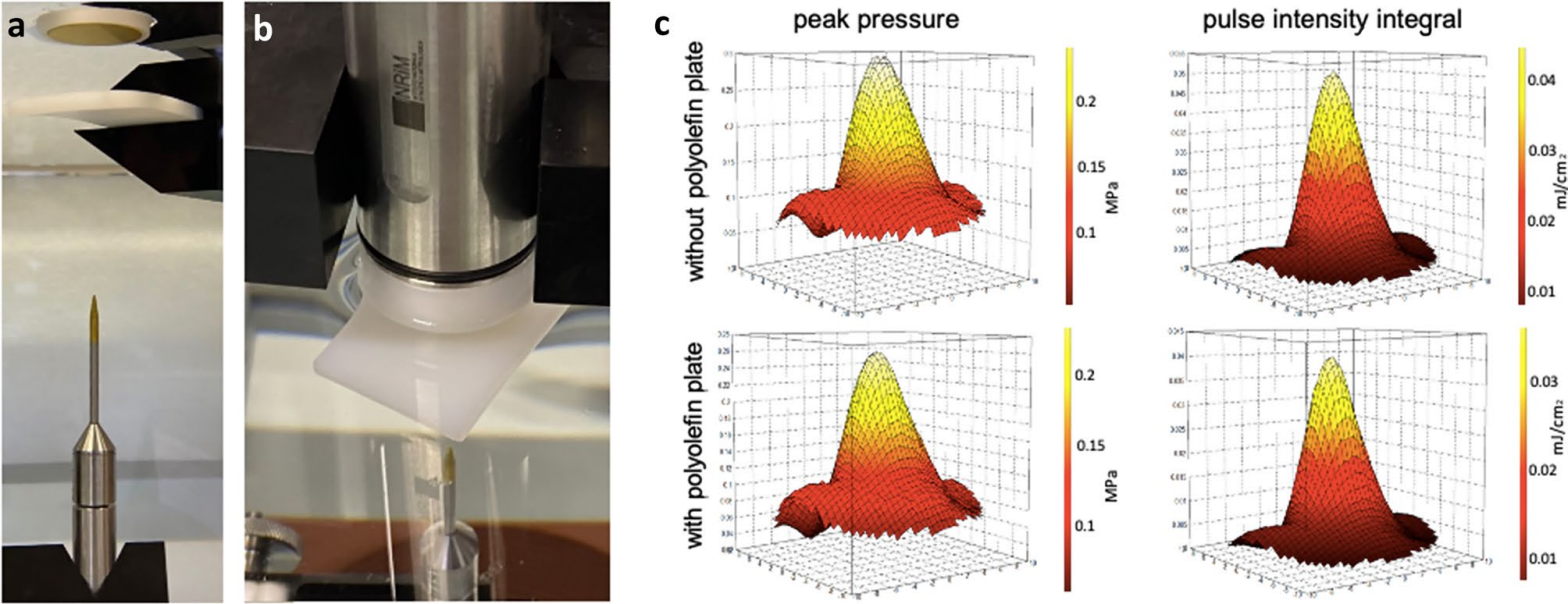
Acoustic characterization of the ultrasound-transparent polyolefin plate. (a,b) Measurements setup: (a) from top to bottom: surface of TRA08 transducer, polyolefin plate, HNC-0400 hydrophone. (c) Comparisons between two-dimensional measured array pressure (left panels) and energy (right panels), with polyolefin plate placed at 15 mm from the transducer surface (lower panels) and without polyolefin plate (upper panels).
